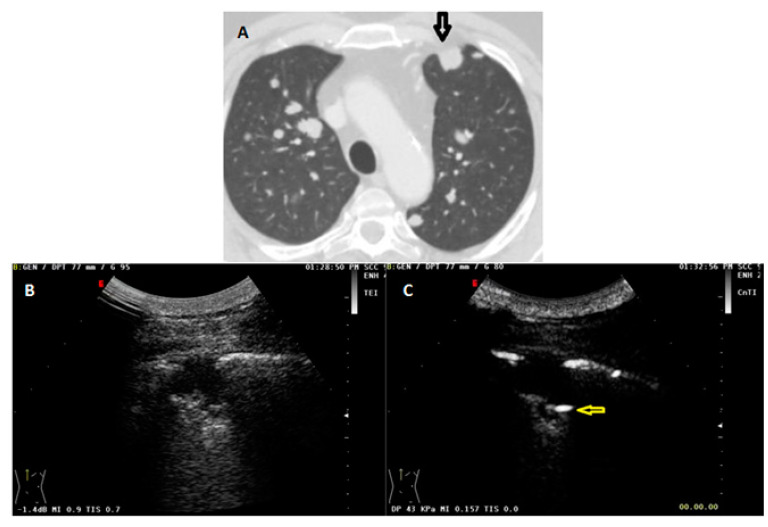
(A) Axial chest CT scan (lung window) showing a subpleural malignant pulmonary lesion in the anterior segment of the left upper lobe (black arrow). (B) The corresponding US B-mode scan showed a subpleural hypoechoic lesion with a hyperechoic spot within. (C) In the US scan with contrast setting (mechanical index: 0.157), but without the administration of contrast agent, seems to be even enhanced (yellow arrow).
